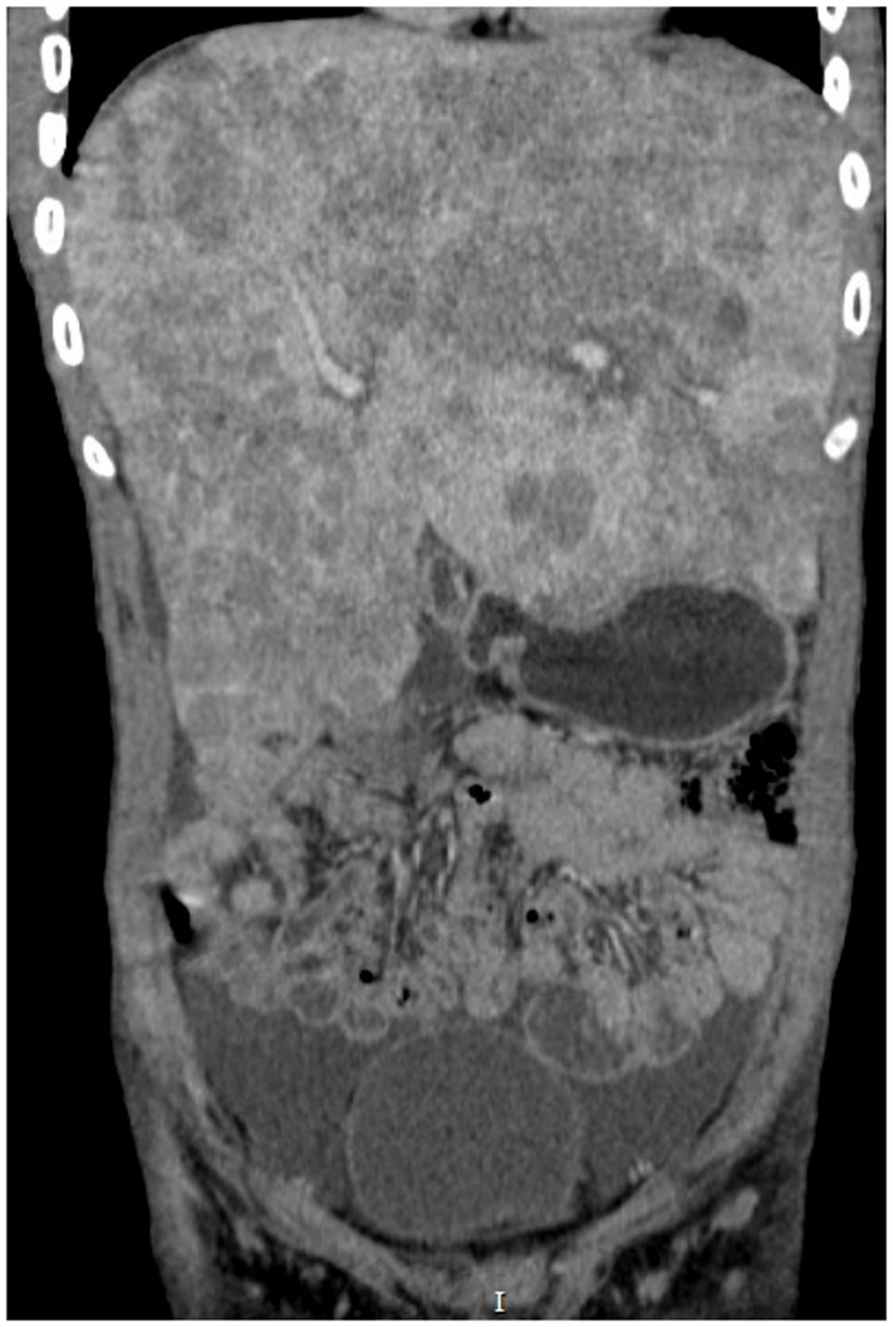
Abdominal CT in coronal cut showing the massive hepatomegaly.
